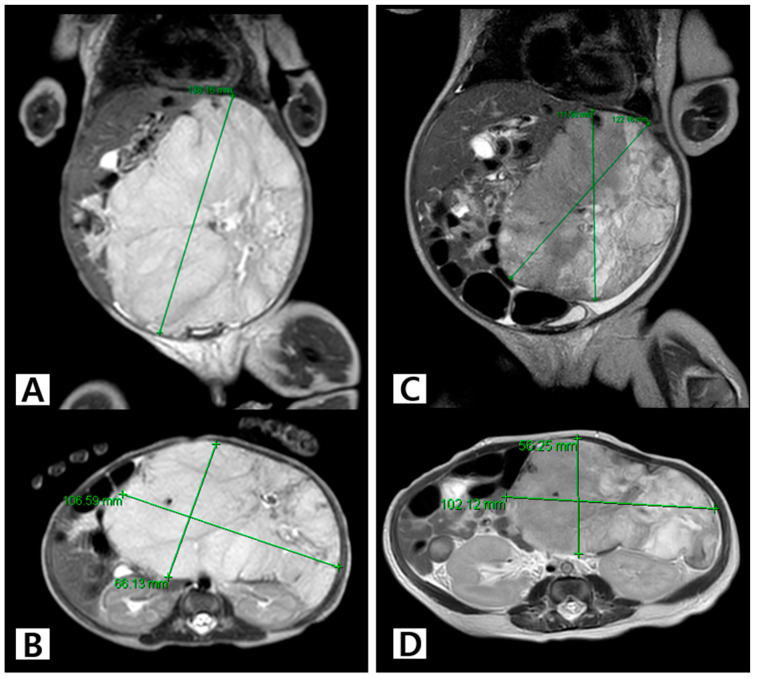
The MRI scans of Patient 11, who underwent step-by-step medical treatment and surgical resection, which led to a complete resolution of the tumor. (A,B) Coronal and axial MRI performed on the fifth day of life shows a 14 × 10 × 6.5 cm sized, well-defined, and lobulated mass suggestive of HH. The patient presented with abdominal distension, vomiting, and respiratory difficulty. (C,D) Coronal and axial MRI performed at the age of 6 weeks shows a partial reduction in the mass without clinical improvement in symptoms, after hepatic artery embolization and the administration of oral propranolol, prednisolone, and sirolimus. Abbreviations: HH, hepatic hemangioma; MRI, magnetic resonance imaging.
